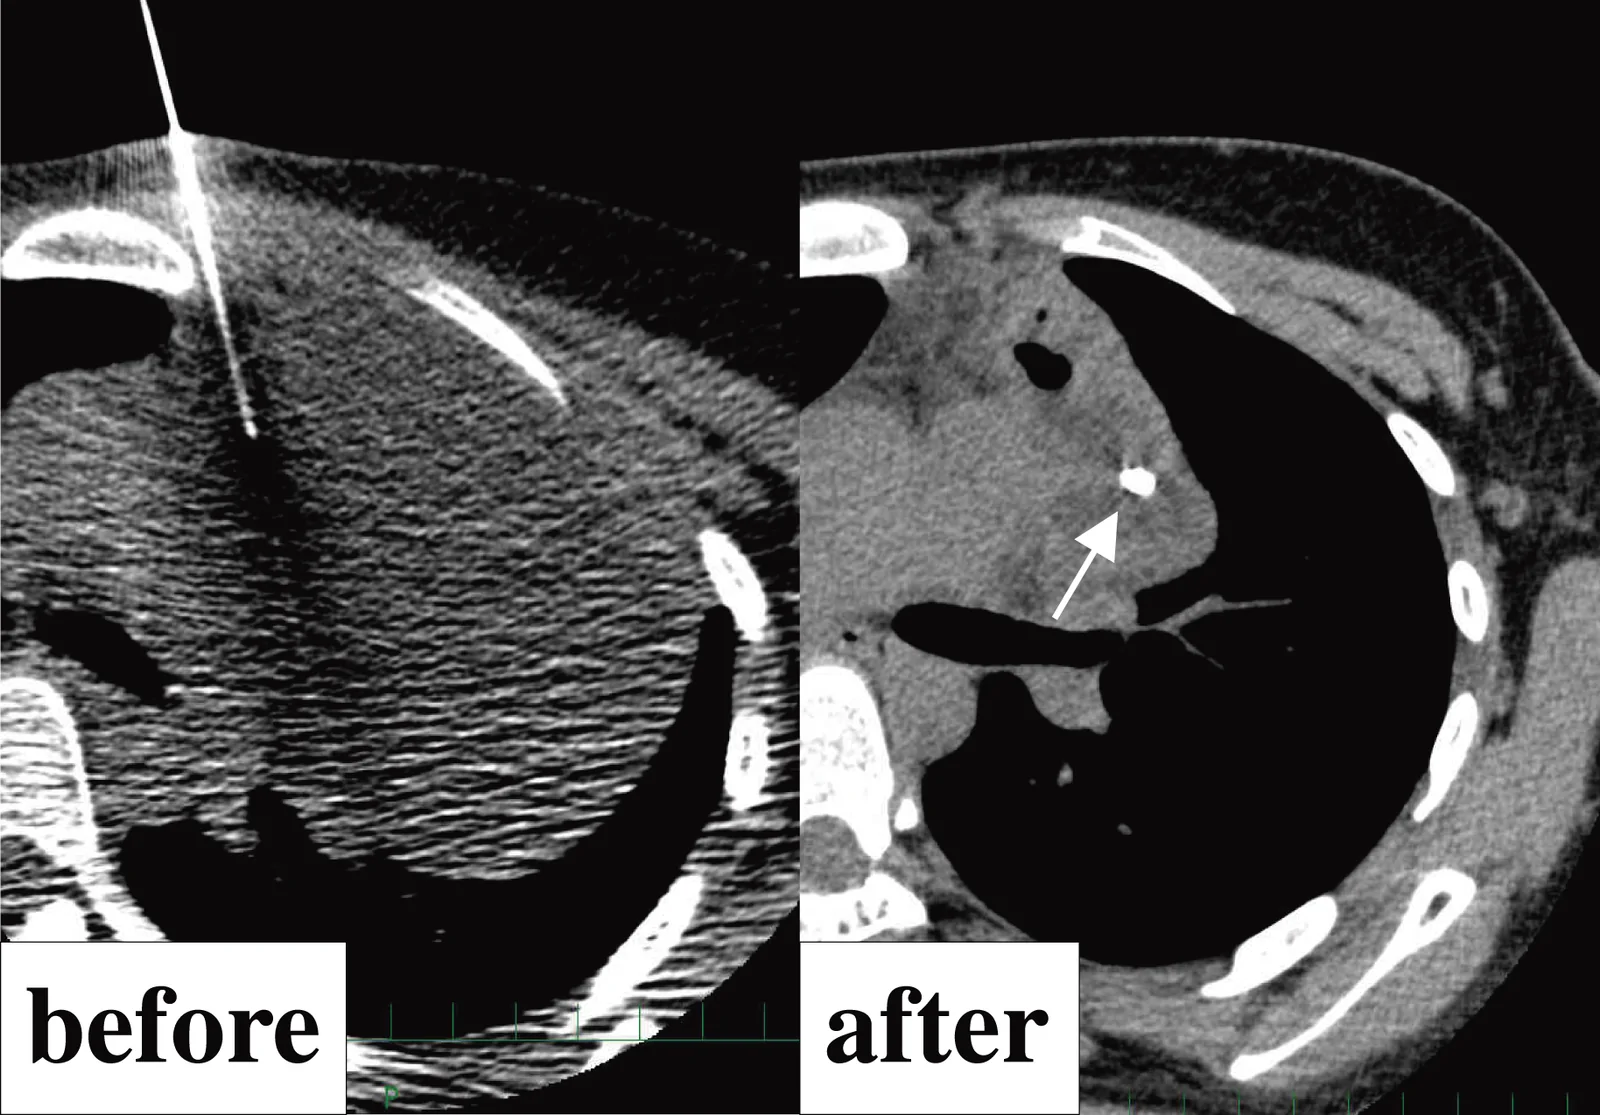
Computed tomography before and after CT-guided transmediastinal drainage. A marked reduction in tumor size is observed after drainage, with expansion of the intrathoracic space. The arrow indicates the indwelling drainage catheter.
